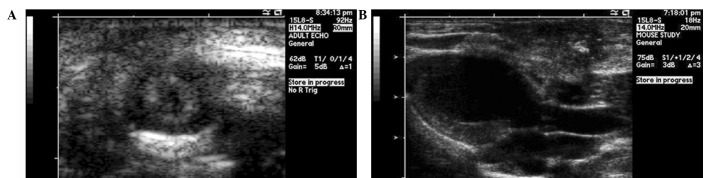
Echocardiography of (A) the parasternal short axis view and (B) the parasternal long axis view.
